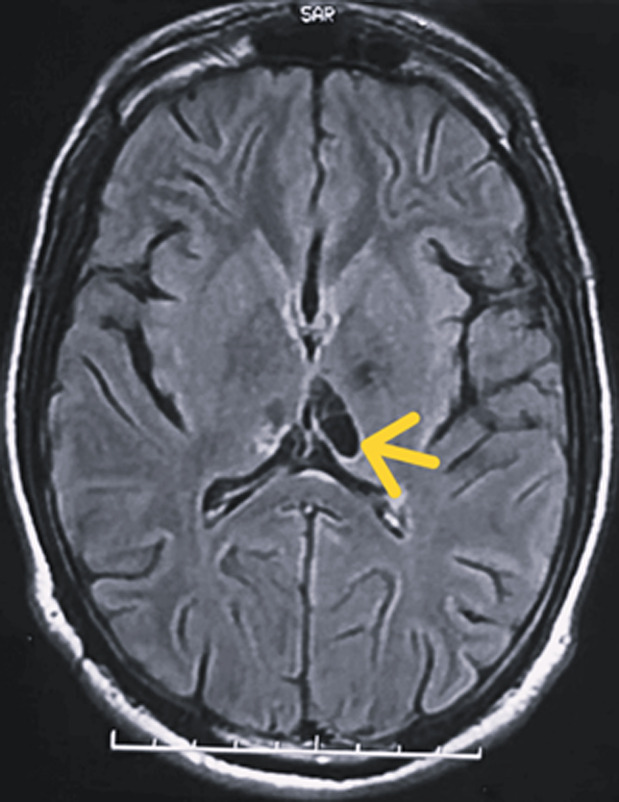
axial brain magnetic resonance image showing post-treatment gliotic changes: axial fluid-attenuated inversion recovery magnetic resonance sequence obtained after radiotherapy demonstrating gliotic signal changes and collapse of the third ventricle (yellow arrow)
